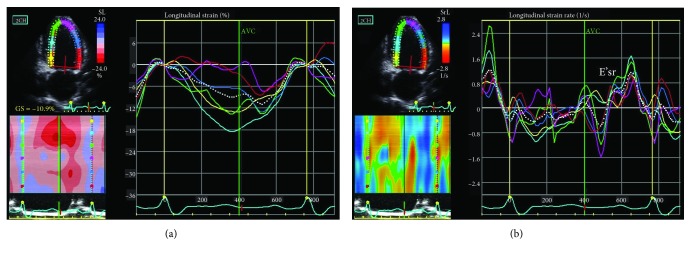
A representative example of measurements of global longitudinal strain (a) and the early global diastolic strain rate (b) from the curves of longitudinal strain and the strain rate of six segments of the left ventricle in the apical two-chamber view. AVC: aortic valve closure; E'sr: early global diastolic strain rate.
